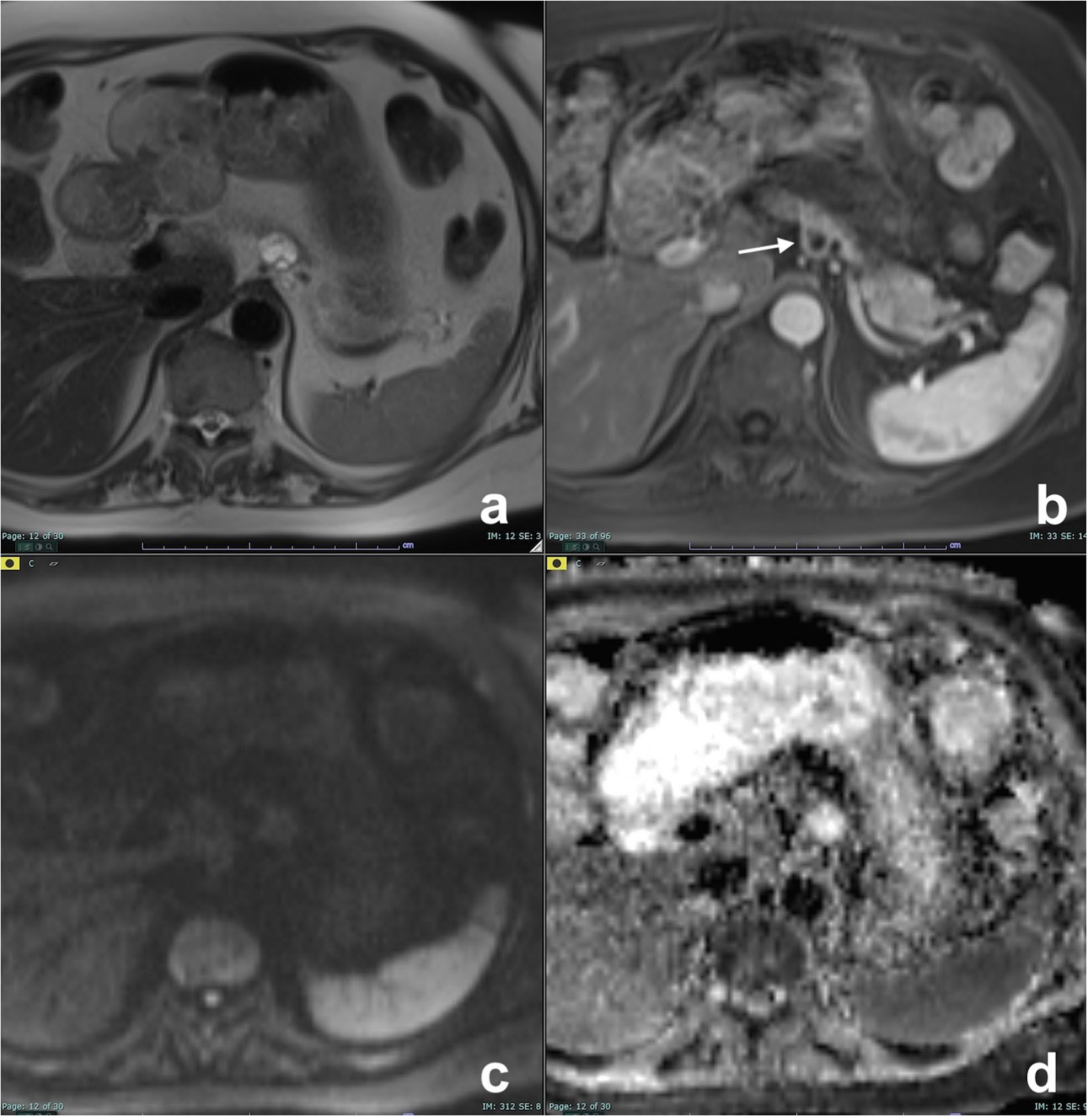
Cystic PNET. On T2w (a), a small hyperintense lesion with tiny septa is appreciable in the body of the pancreas. At T1w (b), during injection of paramagnetic contrast media an intense rim enhancement can be appreciable in the arterial phase (arrow). On DWI (c), no restricted diffusion can be appreciated and the lesion appears hyperintense at ADC map (d). After surgical removal, a G1 PNET was diagnosed at pathology
